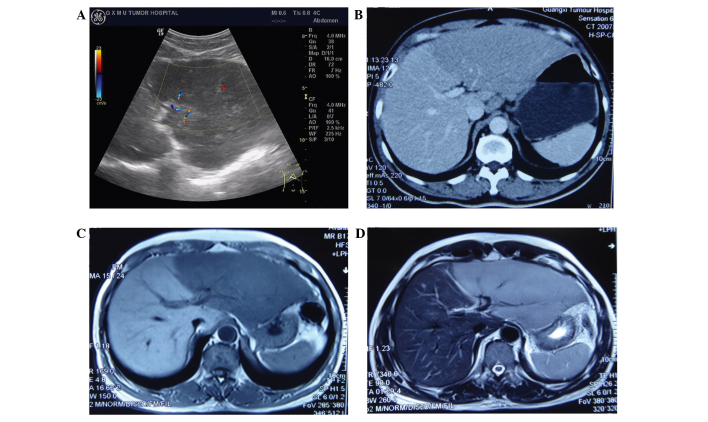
Imaging studies of the left lobe of the liver by (A) ultrasound examination, (B) computed tomography, and (C) T1-weighted imaging (WI) and (D) T2WI by magnetic resonance imaging.
